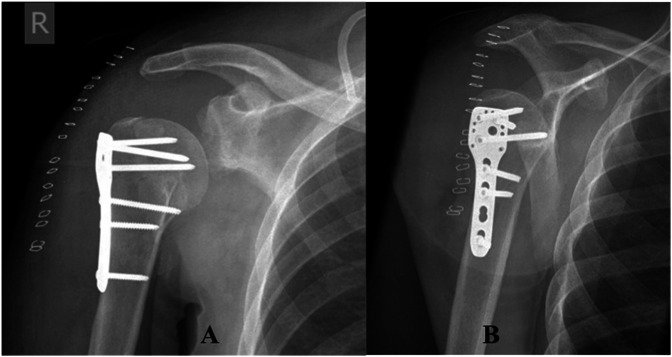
Anteroposterior (A) and lateral (B) radiographs showing a good reduction of the right shoulder using a 4-hole humeral locking plate
